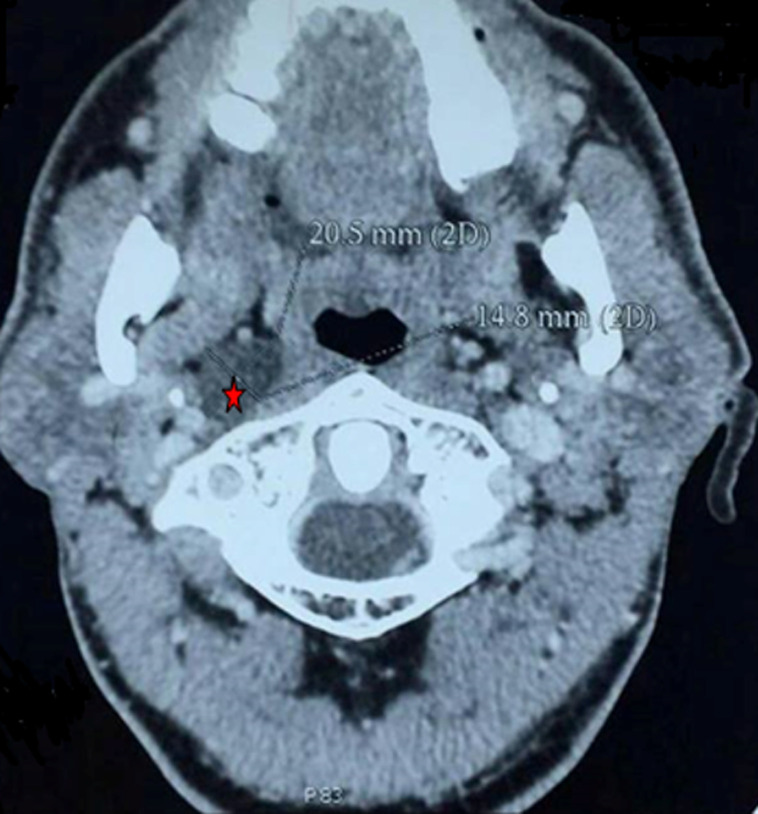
axial post-contrast CT image showing a non-enhanced cystic mass in the right retropharyngeal space
